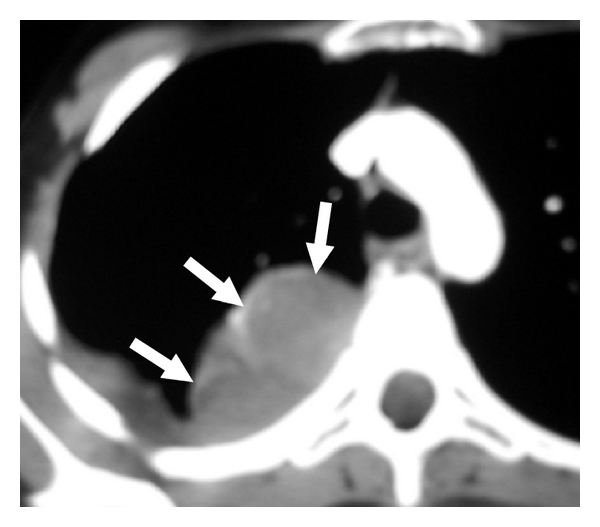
Axial contrast-enhanced CT of a 17-year-old girl demonstrates a heterogeneous soft tissue mass in the right hemithorax (arrows) that abuts the costal and vertebral pleura, with no frank evidence of bony invasion. A small right pleural effusion is also present. There is no visible tumor invasion into the spinal canal. Biopsy of the lesion confirmed metastatic Wilm's tumor.
